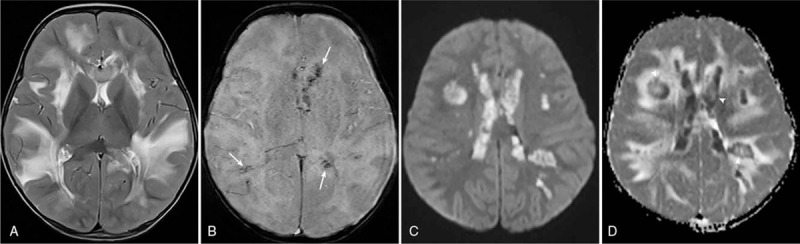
Initial neuroimaging findings of Case 1 presented with fever and seizure after parainfluenza – a virus and bocavirus infection. (a) Axial T2-weighted images demonstrate widely distributed T2 hyperintensity in both the cerebral hemispheres predominantly involving the white matters while sparing both the basal ganglia and thalami. (b) Petechial hemorrhages are demonstrated on SWIs (arrows). (c) On DWIs (*b* = 1000 s/mm^2^), lesions show multifocal diffusion restrictions in both cerebral white matters. (d) On the ADC map, the lesions show “tricolor pattern” or “target appearance” (arrow heads), which represent central necrosis and hemorrhage, cytotoxic edema of the middle portion, and outer vasogenic edema. ADC = apparent diffusion coefficient, DWI = diffusion-weighted image, SWI = susceptibility-weighted image.
